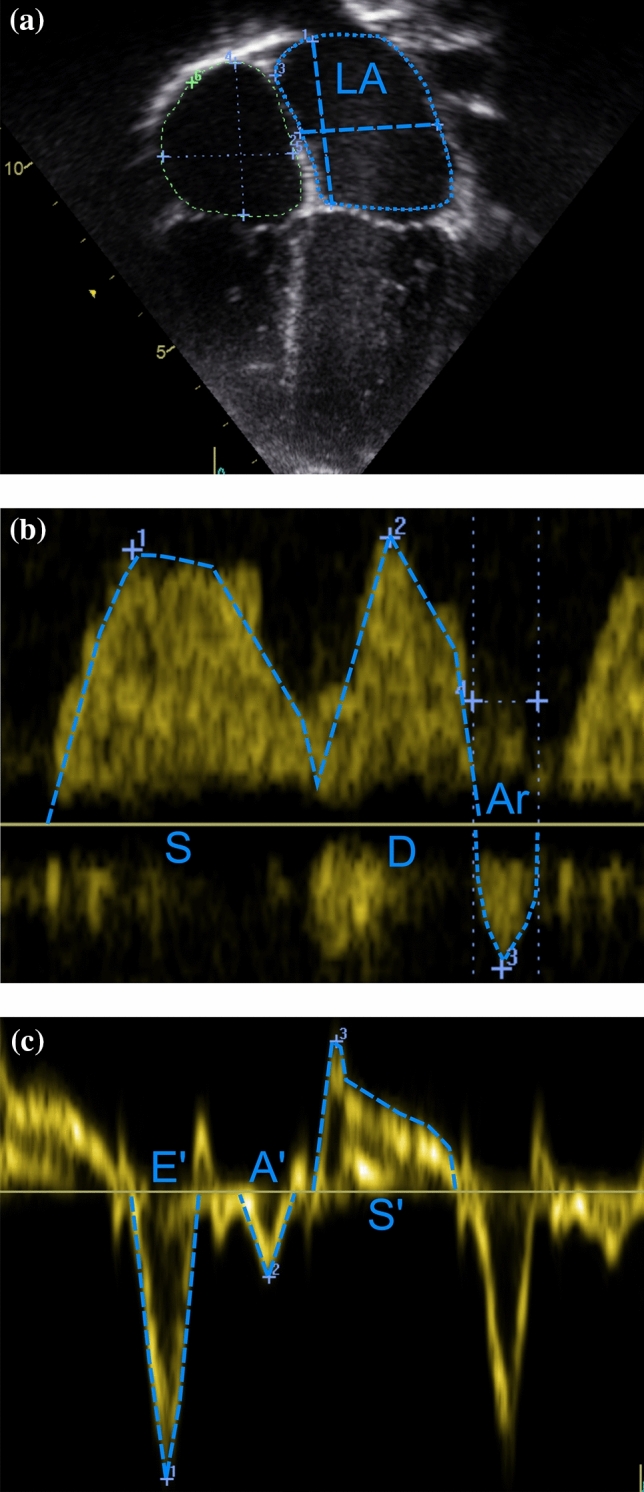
Assessment of left ventricular diastolic function: a Left atrial volume calculation with biplane area-length method (apical four-chamber view at ventricular end-systole showing left atrial major-axis length and left atrial planimetered area). b Pulmonary venous systolic-to-diastolic peak velocity ratio (S/D), pulmonary venous A wave reversal (Ar) amplitude and duration with Doppler. c Mitral lateral peak early and late diastolic, and systolic tissue velocities (E′, A′, S′) with Tissue Doppler Imaging.
